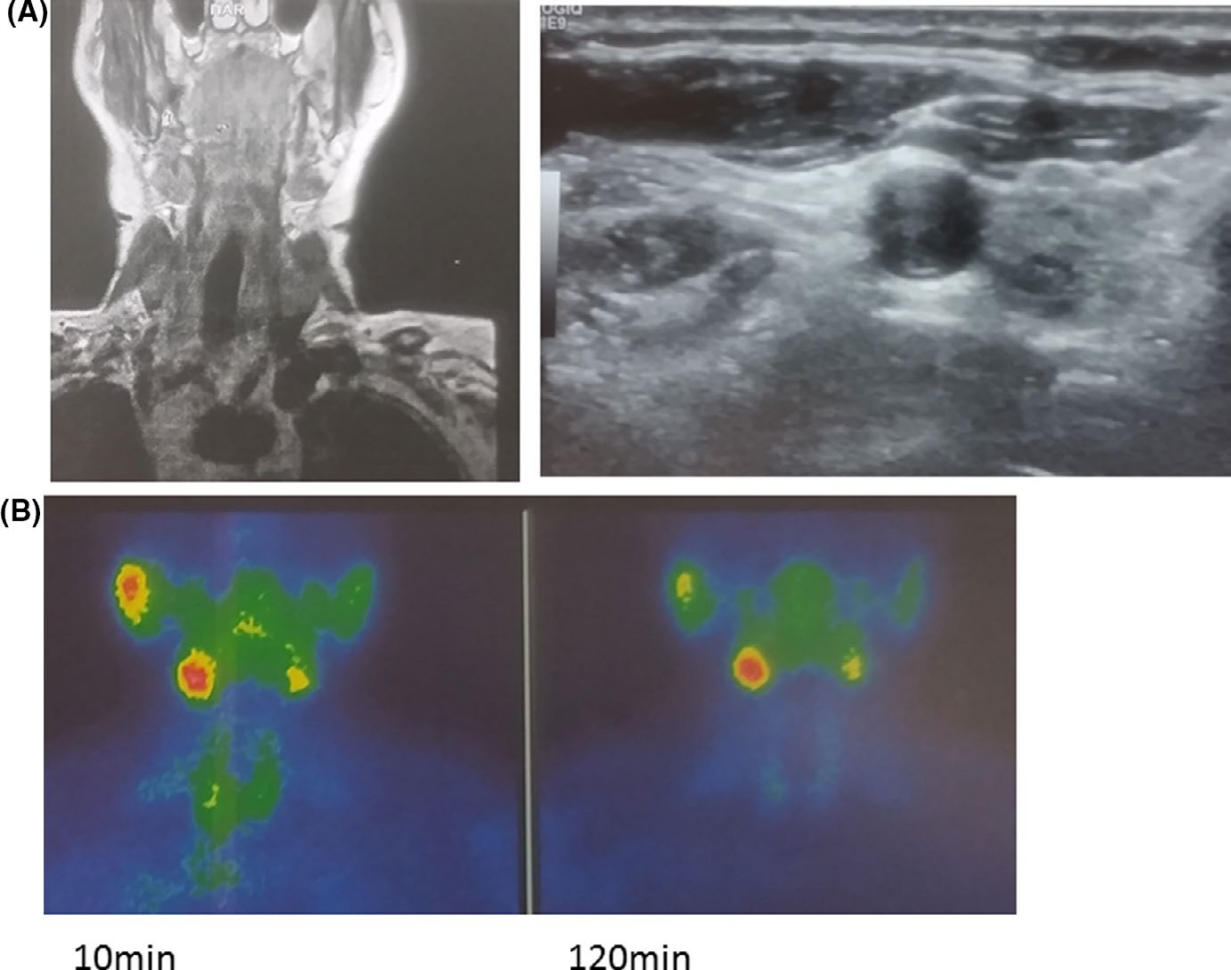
A, Neck CT scanning with IV contrast and transverse neck U/S showing no pathologic findings from the parathyroid glands. B, Sesta‐MIBI scanning 10 min and 120 min after the injection of the radiotracer without pathologic focal uptake
